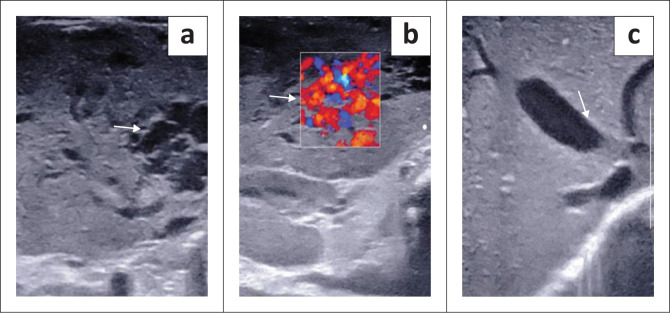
A 4-month-old female with congenital haemangioma diagnosed antenatally. Transverse greyscale ultrasound (a) with colour Doppler of the liver (b) shows an ill-defined iso- to hypoechoic lesion (arrow in a) with cystic spaces corresponding to vascular channels on colour Doppler in the left lobe of liver (arrow in b) and an ectatic middle hepatic vein (arrow in c).
